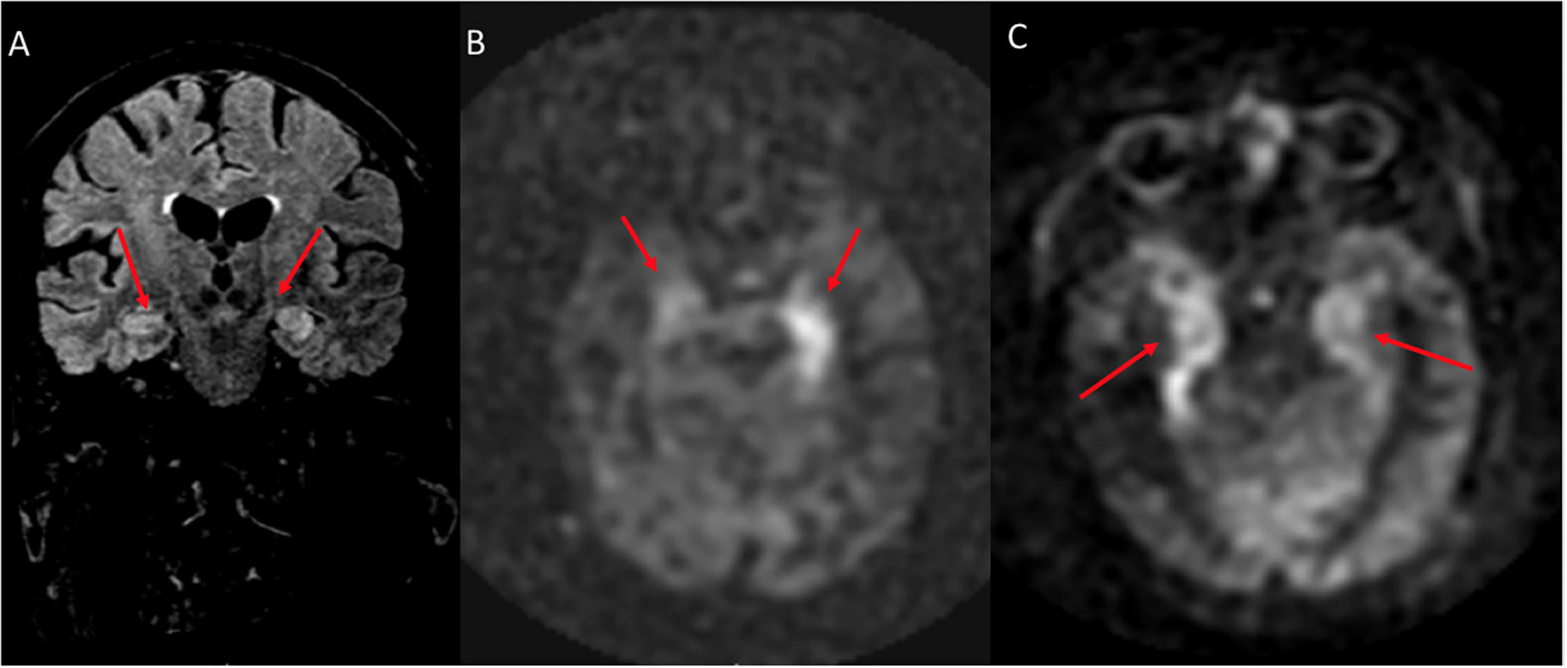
A 76 year old male with subacute cognitive decline. (A) Coronal FLAIR demonstrates hyperintense signal in the left greater than right hippocampus and amygdala. (B) ASL shows hyperperfusion in the left greater than right hippocampi. (C) ASL demonstrates bilateral involvement.
